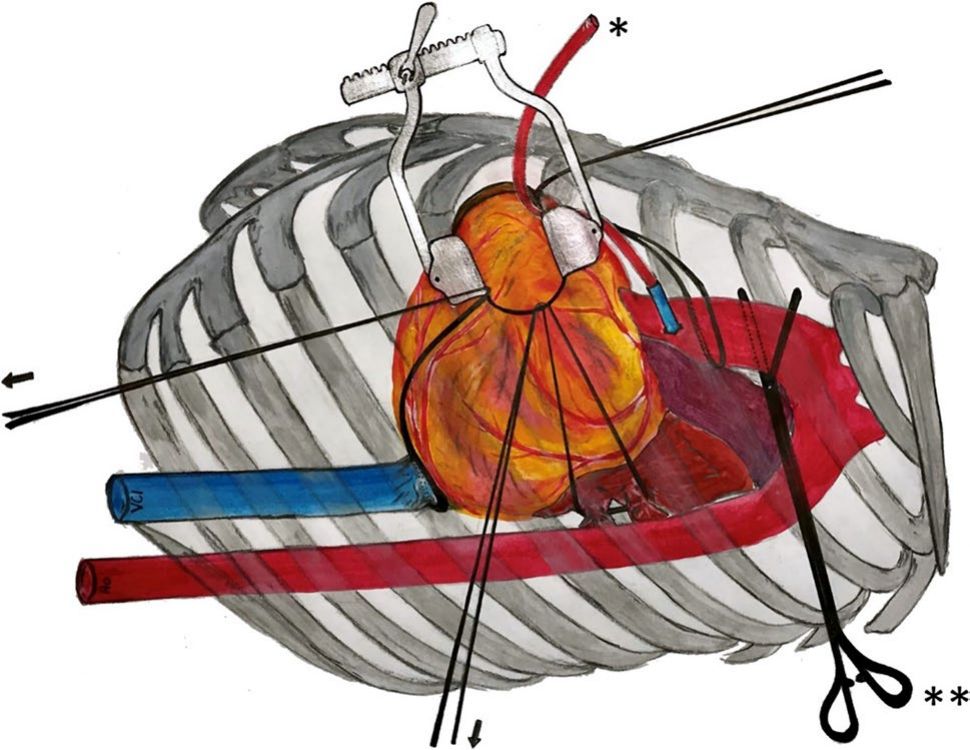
After induction of cardioplegic through cardioplegia line (*), cardiac arrest with transthoracic aortic cross-clamping in the third intercostal space in the left anterior axillary line () and peripheral CPB cannulation, the heart can be rotated and moved toward the anterior incision using tapes (arrows) encircling aorta, inferior caval vein and left pulmonary veins for exposure of coronary targets at lateral and inferior left ventricular wall. *CPB* cardiopulmonary bypass
